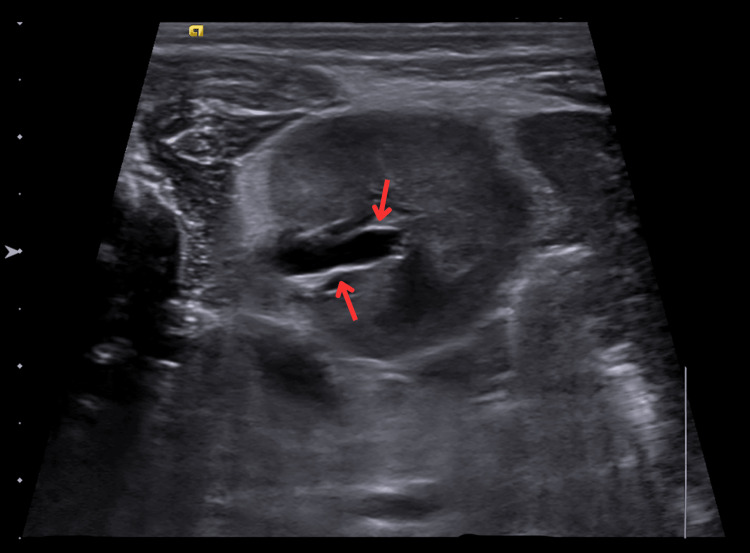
Renal ultrasonography showing urothelial thickening in the infant
